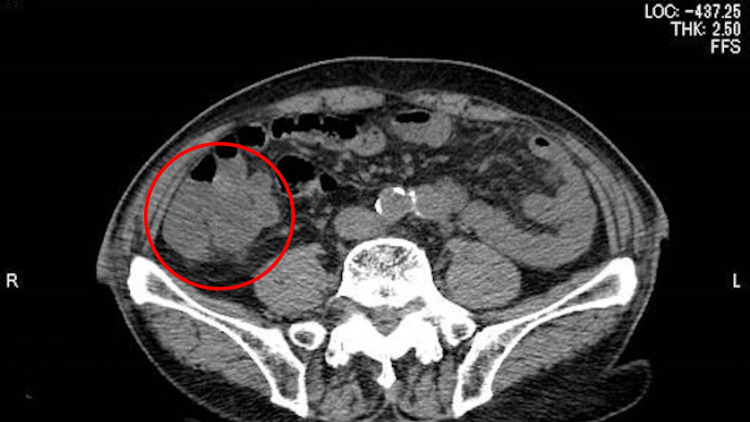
The abdominal computed tomography showing the edematous lesions in the small intestine and colon (red circle).
